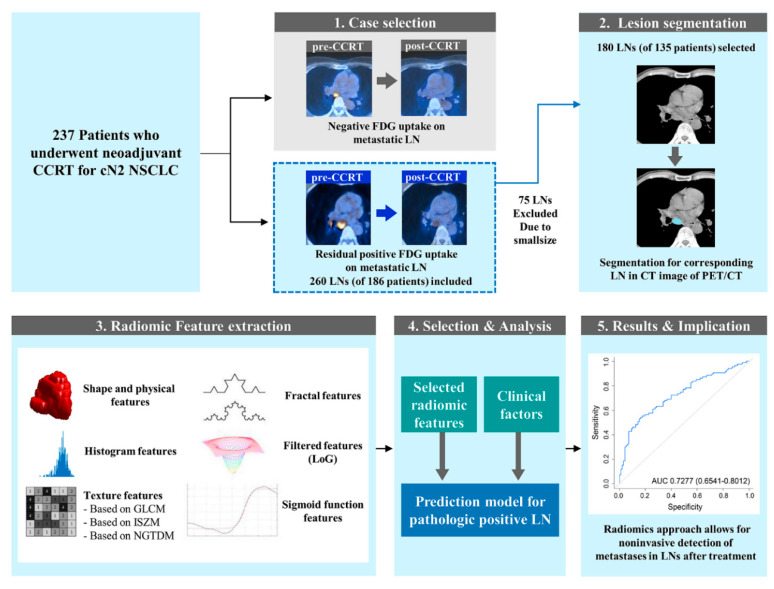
Overall processing steps. In the case selection step, the presumed metastatic lymph nodes upon pre-concurrent chemoradiotherapy (CCRT) positron emission tomography-computed tomography (PET-CT) with abnormal residual 2-[fluorine-18]fluoro-2-deoxy-d-glucose uptake in post-CCRT PET-CT were selected. The region of interest of the whole lymph node was independently segmented in the CT component of PET-CT. In this study, a total of 252 features were extracted for the ROI from the raw imaging data. The features belonged to one of the following six categories: (1) shape and physical-based, (2) histogram-based, (3) texture-based, (4) fractal-based, (5) filter-based, and (6) sigmoid function-based. The final step of the study was to construct a prediction model for pathologic positive lymph nodes incorporating selected radiomics features and clinical factors.
